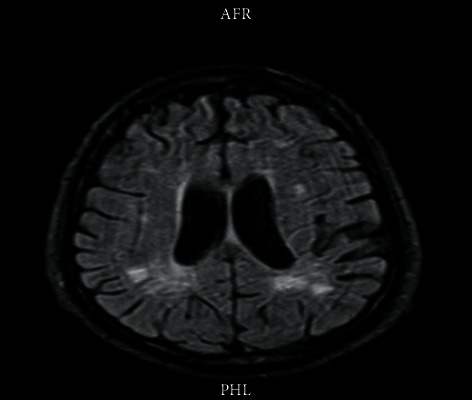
Brian MRI showing bilateral cerebral subcortical, deep white matter, and centrum semioval bright signal foci in *T*2/weighted images.
